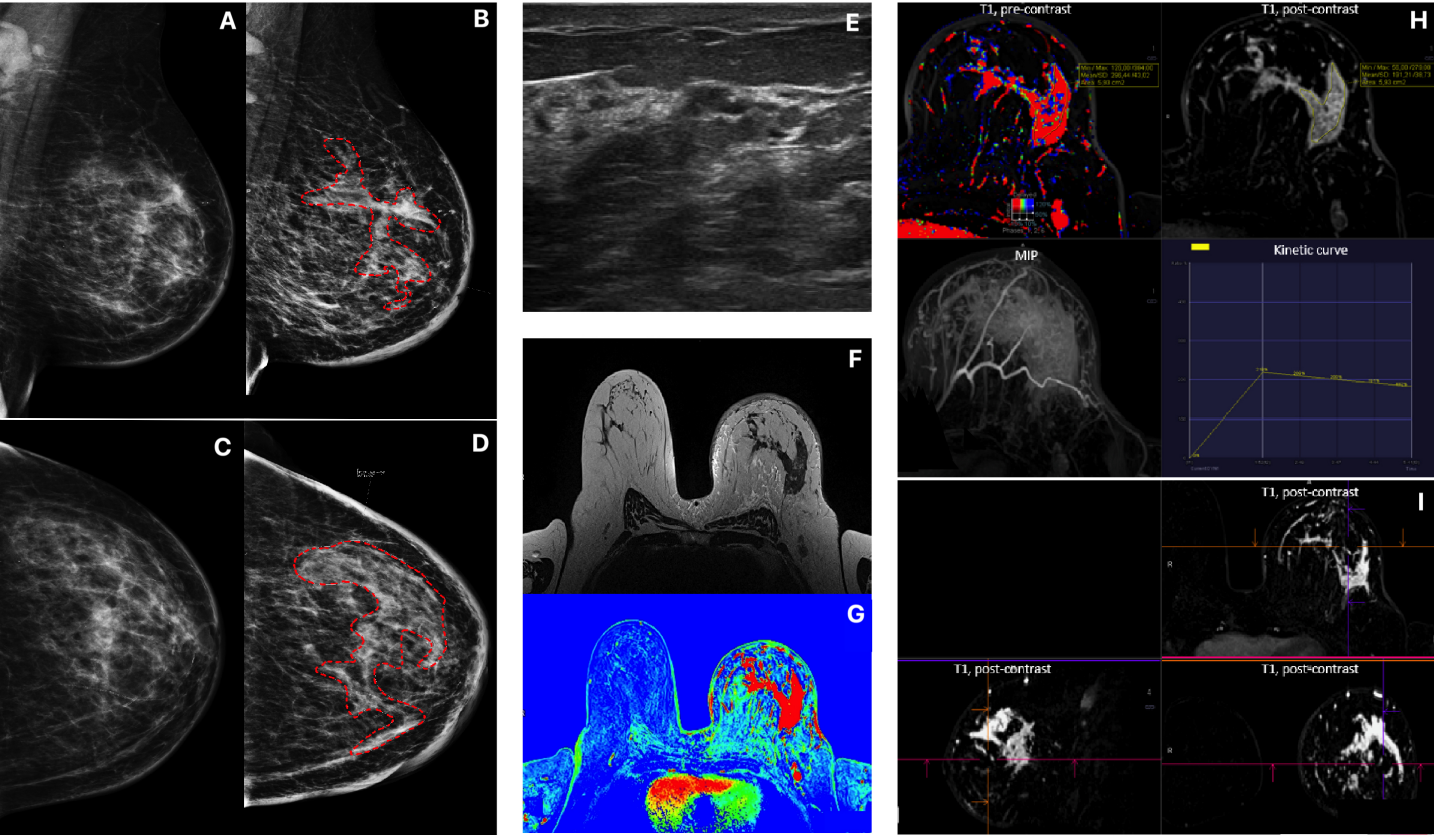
Patient 2, Multimodality presentation of Lobular Breast Cancer, left breast: (A, B) Mammography, [(A) MLO and (B) CC] asymetric density cancer, cancer marked with red dotted line. (C, D) Mammography, [(C) MLO and (D) CC] the same Patient after 4 months, cancer marked with red dotted line. (E) ultrasound- not circumscribed, hypoechoic region. (F) MRI T2 TSE - non-mass enhancement. (G) MRI T1 fl3d dynamic PEI. (H, I) MRI - diffused, non-mass enhancement with heterogenous enhancement [(H) T1 pre contrast; T1 post contrast; MIP and wash out kinetic curve; (I) 3D T1 post contrast].
